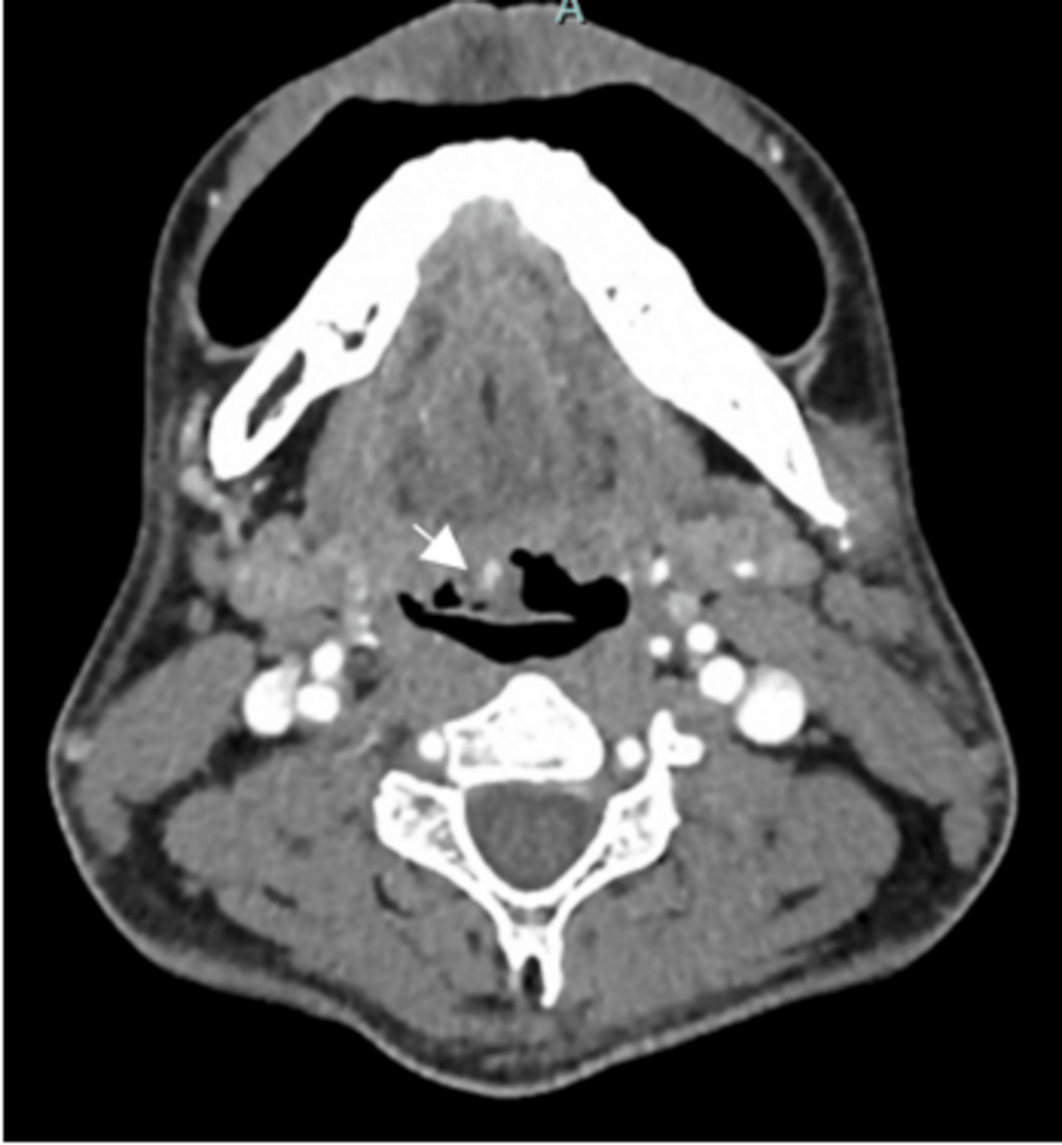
Axial CECT neck showing irregular exophytic enhancing lesion at the right base of the tongue (white arrow). There is hyperdensity at the right base of the tongue to suggest calcification. It abuts the epiglottis. CECT: contrast-enhanced computed tomography
